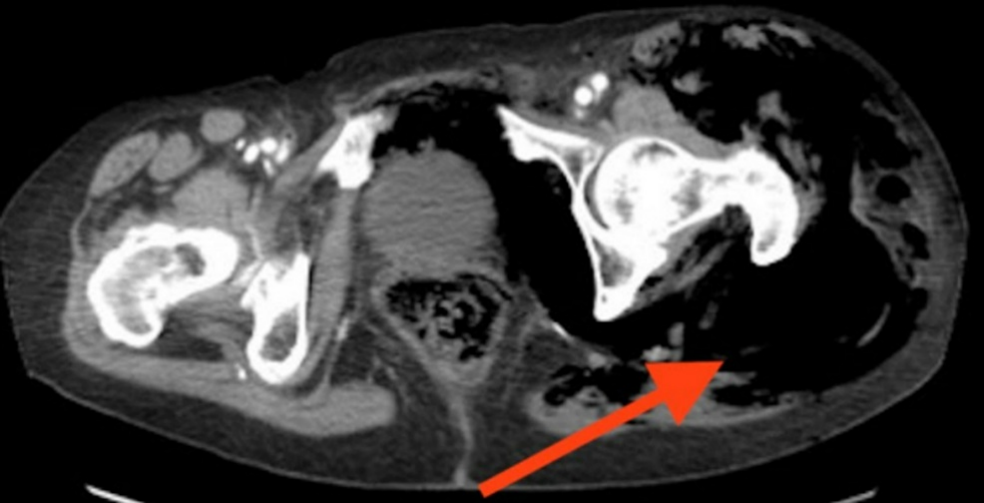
Computerized tomography (CT) pelvis transverse view showing free air in the left gluteal area (red arrow)
